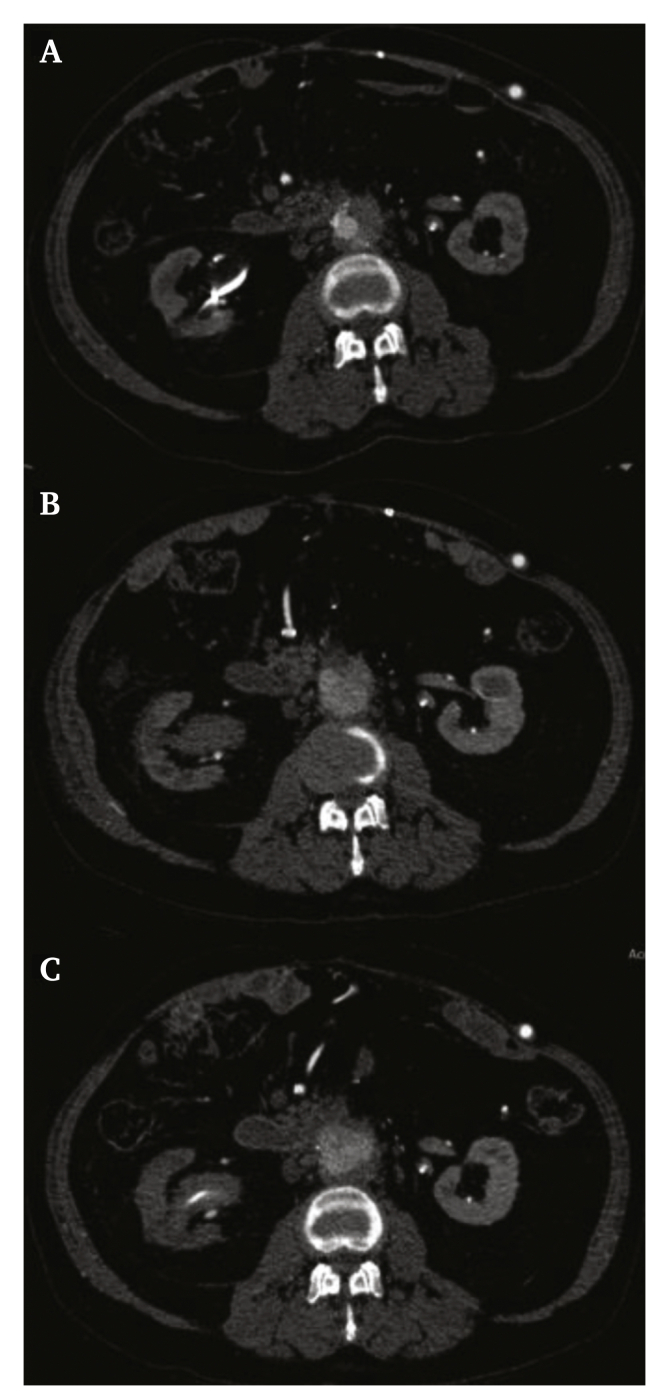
CTA demonstrating progressive dilatation of the aortic stump. CTA demonstrating persistent infection of the aortic stump, with the Amplatzer® Vascular Plug in situ. Also, it shows progressive dilatation of the aortic stump without signs of acute rupture. The upper, middle and lower images (A, B and C) show the progressive enlargement of the aortic stump from day 0 (A) to day 4 (B) and day 11 (C) during admission.
